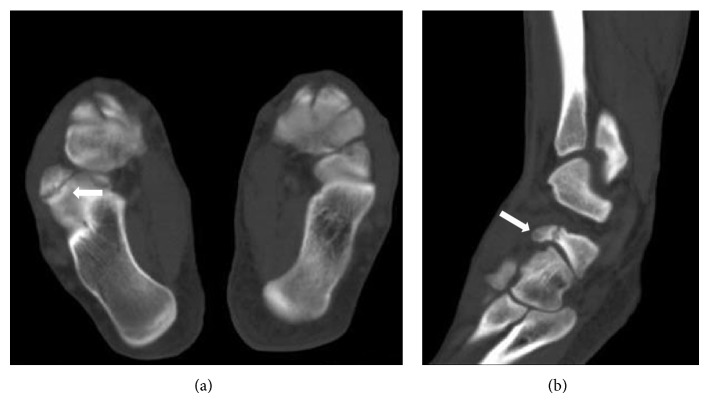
Axial (a) and sagittal (b) reformatted CT images reveal os calcaneus secundarius (white arrows).
